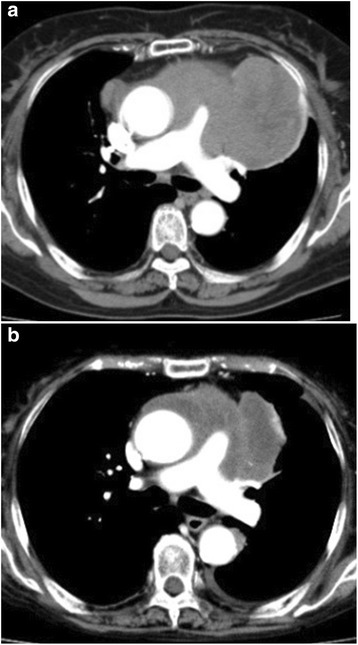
Enhanced computed tomography. a and b are both enhanced computed tomography images. The tumor was well enhanced when it was first found in 2013 (a). The tumor size reduced markedly, and the tumor changed into a poorly enhanced mass after steroid pulse therapy in 2016 (b)
